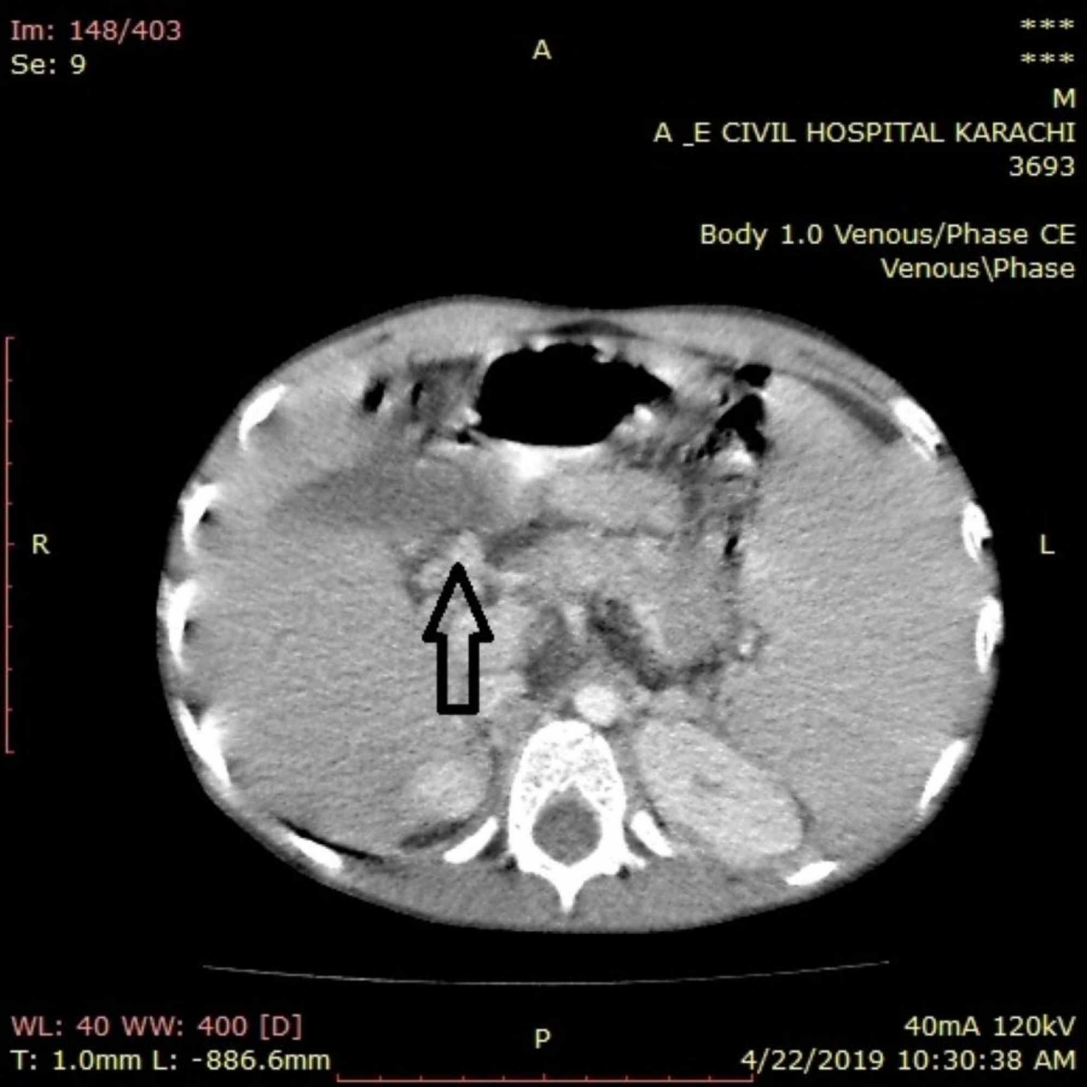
Axial section of CT abdomen showing cavernous transformation of portal vein CT, computed tomography
